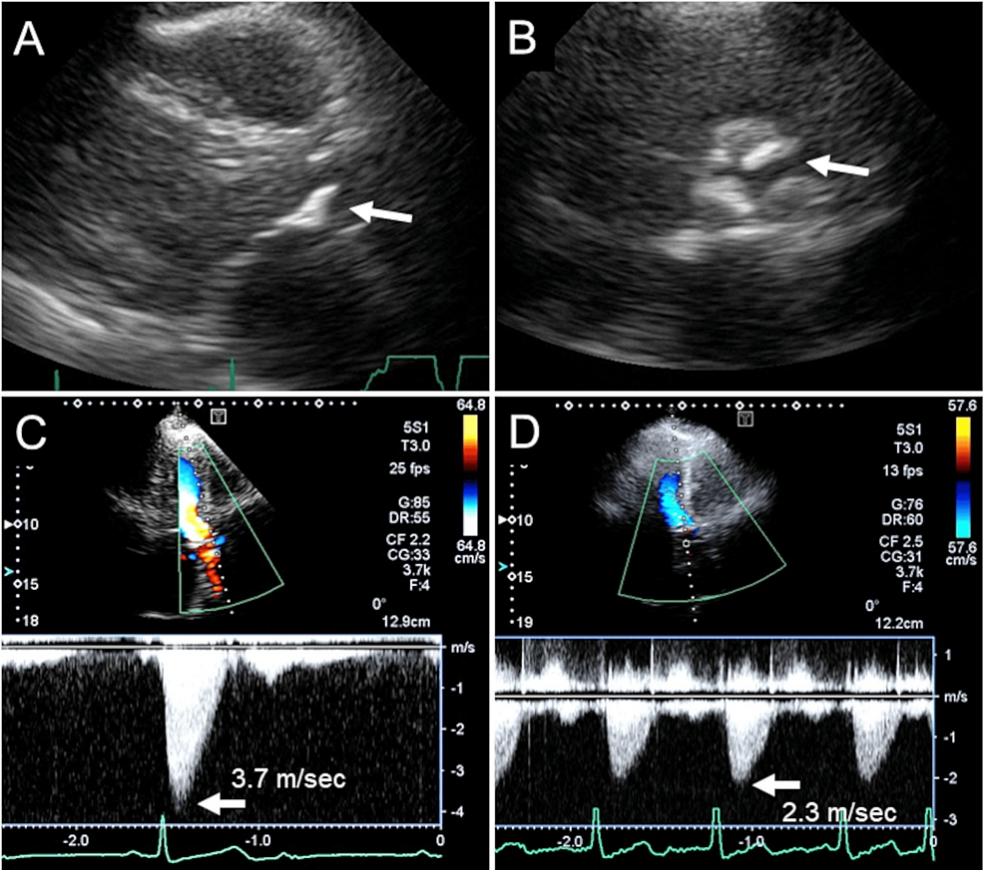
Serial TTEs Initial TTE, which was performed at the time of 2:1 AVB, revealed normal LV systolic function and calcified aortic valve (arrows) (A,B). The severity of AS was considered moderate based on TTE measurements (C). Repeated TTE was performed after the correction of heart rate with pacemaker to evaluate the severity of AS again. The true severity of AS was considered mild under the hemodynamically stable condition (D). TTE: Transthoracic echocardiogram; AVB: Atrio-ventricular block; AS: Aortic valve stenosis; LV: Left ventricular
